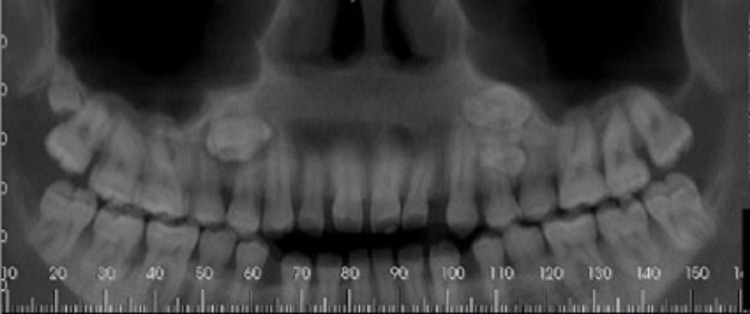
Computed tomography image in panoramic reconstruction showing the presence of four included supernumerary teeth in the region of upper premolars bilaterally and the presence of a fourth molar on the right side.
